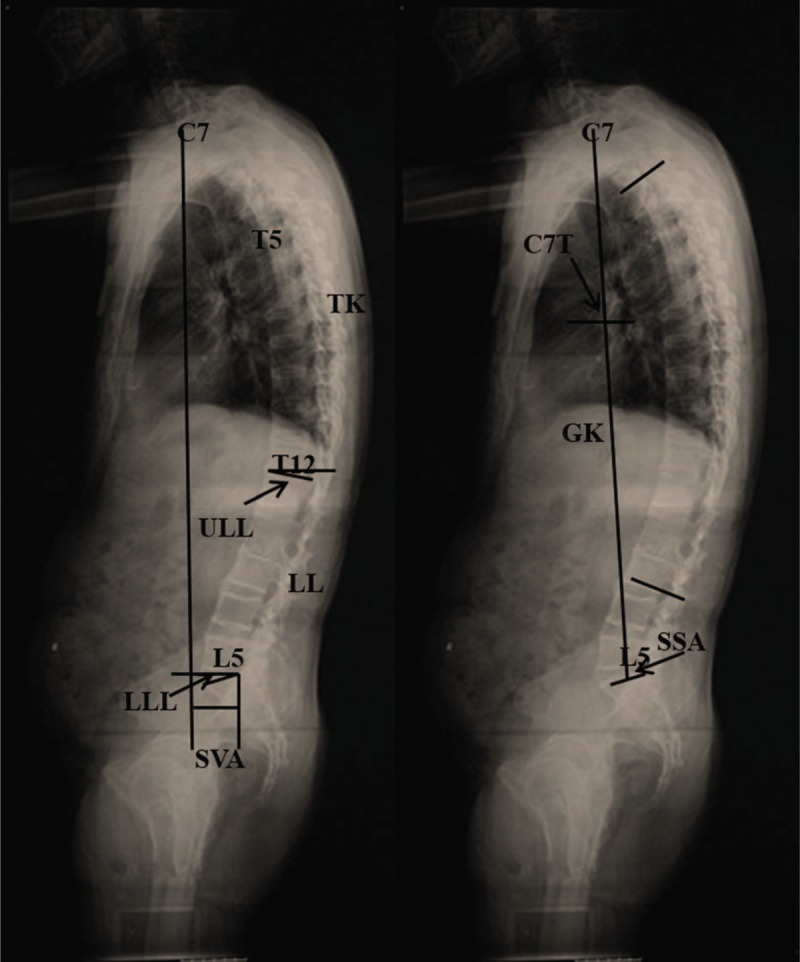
A 46-year-old man with ankylosing spondylitis. Sagittal spinal parameters were measured from the standing lateral radiograph. C7T is the angle between the horizontal plane and the line joining the center of C7 vertebral body and the center of the sacral endplate. SSA is the angle between the sacral endplate and the line joining the center of C7 vertebral body and the center of the sacral endplate. SVA is the distance between the C7 plumb line (C7PL) and the posterior superior corner of S1. GK is the Cobb angle between the upper endplate of the most tilted vertebra cranially and the lower endplate of the most tilted vertebra caudally. TK is the angle between the superior end plate of T5 and the inferior end plate of T12. LL is the angle between the superior end plate of L1 and the superior end plate of S1. ULL is the angle between the tangent line to the vertical axis at the apex of the lumber curve and the superior end plate of L1. LLL is the angle between the tangent to the vertical axis at the apex of the lumber curve and the superior end plate of S1. C7PL = C7 plumb line, C7T = C7 tilt, GK = global kyphosis, LL = lumbar lordosis, LLL = lower arc of lumbar lordosis, SSA = spino-pelvic angle, SVA = sagittal vertical axis, TK = thoracic kyphosis, ULL = upper arc of lumbar lordosis.
